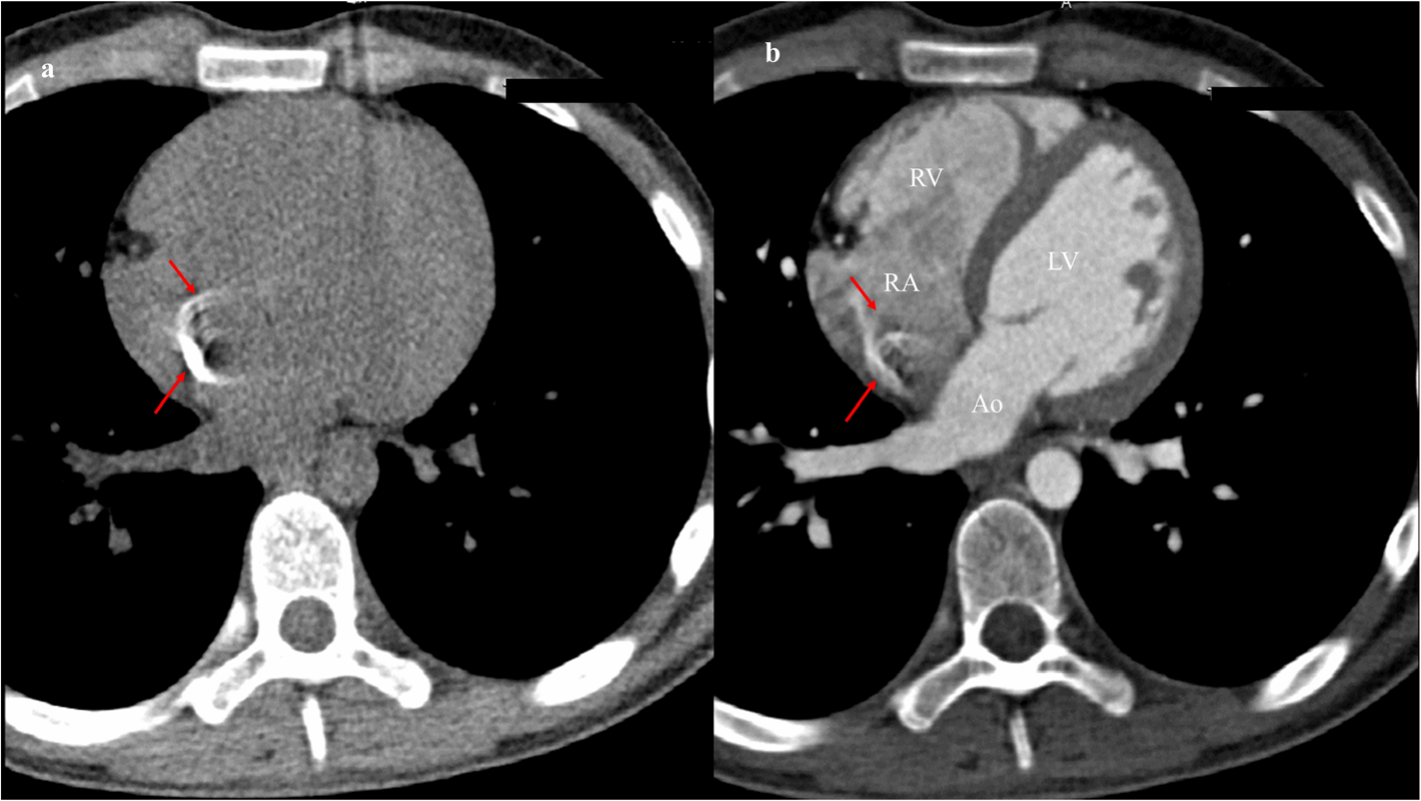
CT showed the large calcified thrombus in the right atrium
